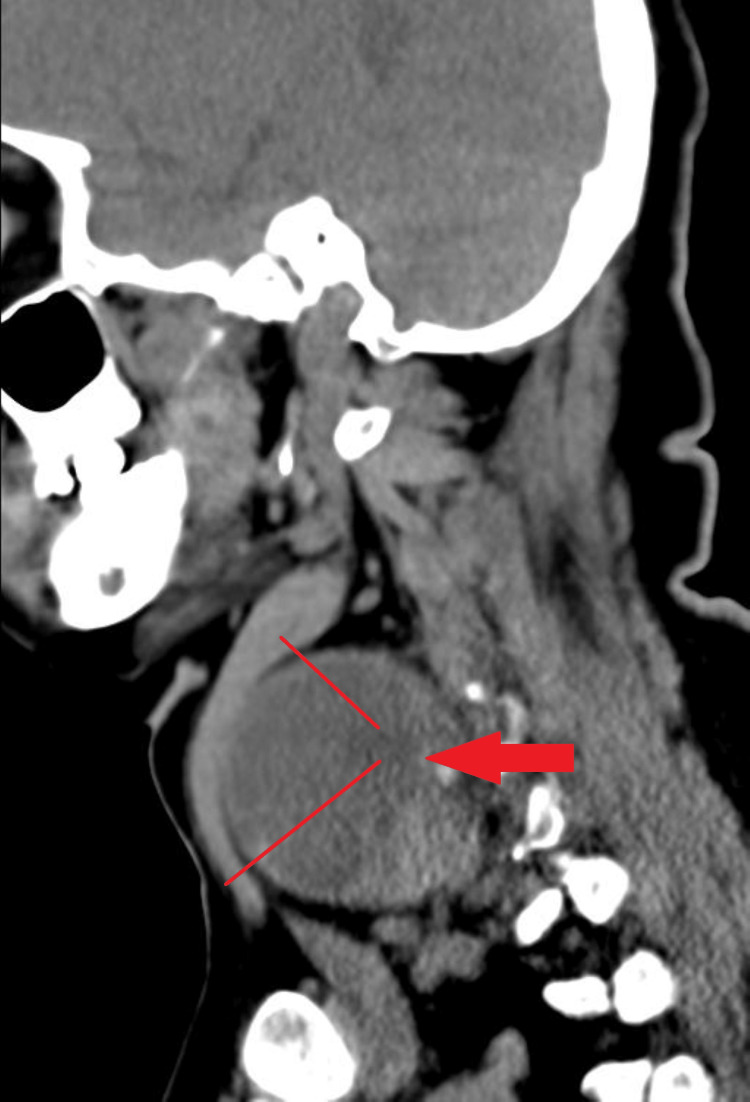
Computed tomography scan image of this patient Red lines and an arrow represent the tumor.
